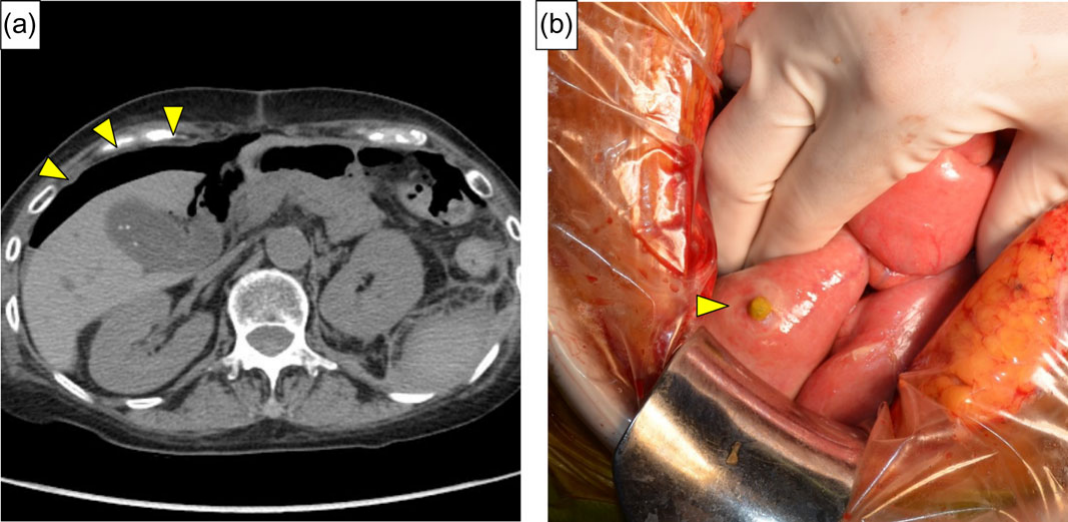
(a) CT showed free air around the right lateral region (yellow arrows). (b) Intraoperative findings showed perforation of the cecum with no obvious masses (yellow arrow).
